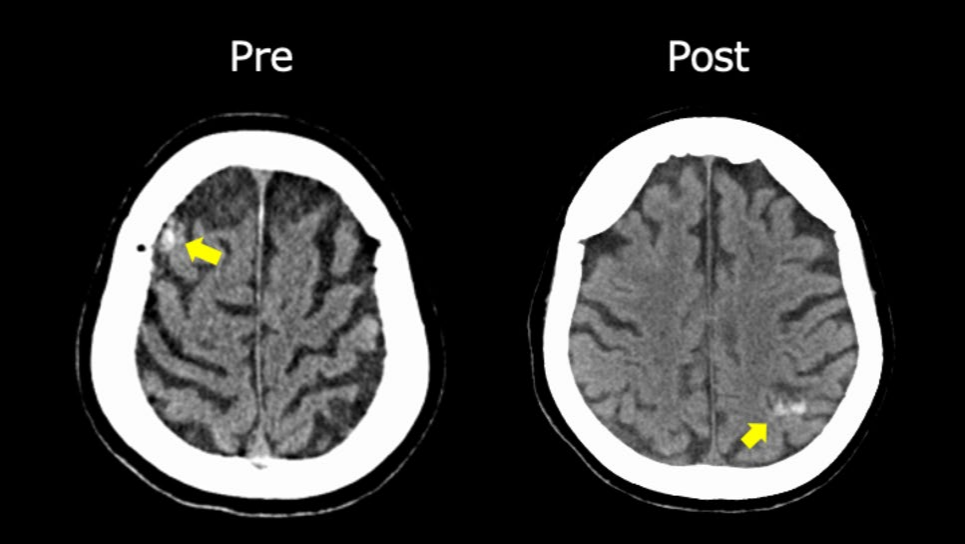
Pre- and postoperative computed tomographic images of patients with postoperative microbleeds. A 83-year-old female had an ectopic microbleeds after valve surgery using nafamostat mesilate. *Yellow arrows* indicate hemorrhagic lesions
